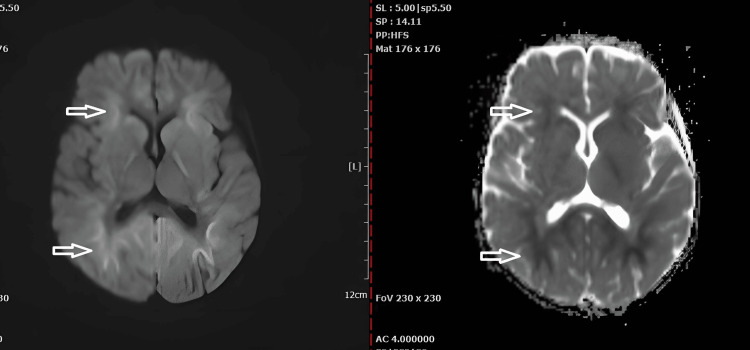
Bilateral symmetrical DWI high and ADC low signal intensity seen at the periventricular white matter (white arrows) shows diffusion restriction. DWI: diffusion-weighted imaging; ADC: apparent diffusion coefficient
